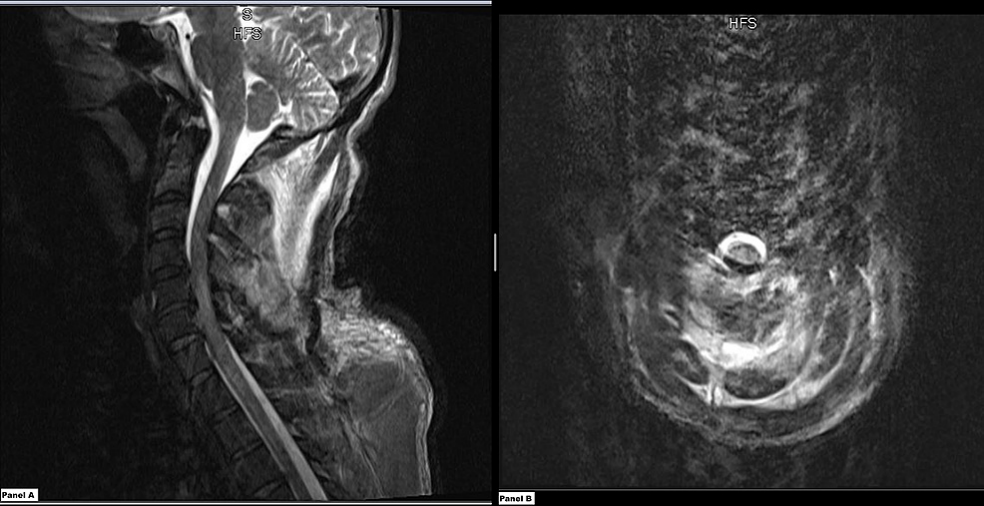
Magnetic Resonance imaging results of the cervical spine Panel A (left): Sagittal section
